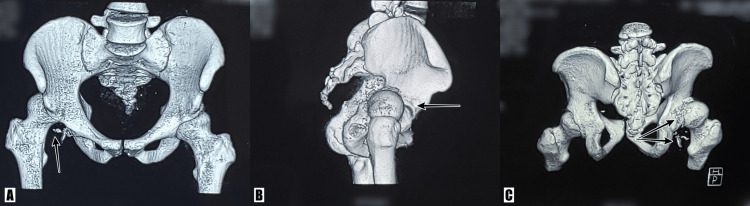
Computed tomography (3D reconstruction) of the pelvis (A, B) A 3D CT reconstruction view showing posterior dislocation of the right hip (arrows). (C) A 3D CT reconstruction view showing right femoral head fracture with multiple fragments (arrows). 3D, three-dimensional; CT, computed tomography
